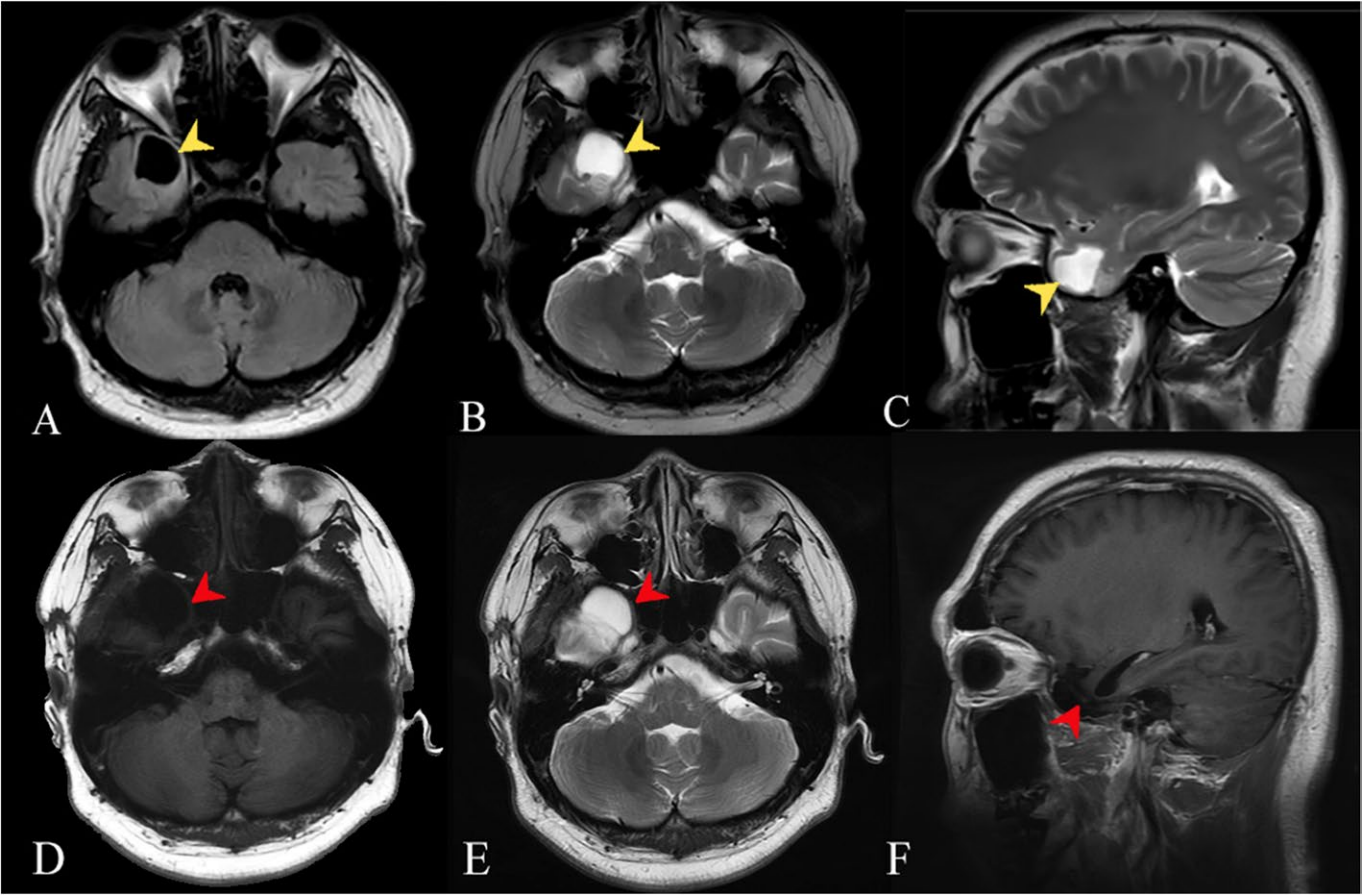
A, B, C The patient’s pre-operative MRI suggested lesion in the right temporal pole with hyperintensity on both T1- and T2-weighted image. C, D, E Follow-up MR images performed six months after the surgery showed increased T1 and T2 signal intensity but no focal enhancing lesion in the right temporal lobe, suggesting post-operative change without residual tumor
